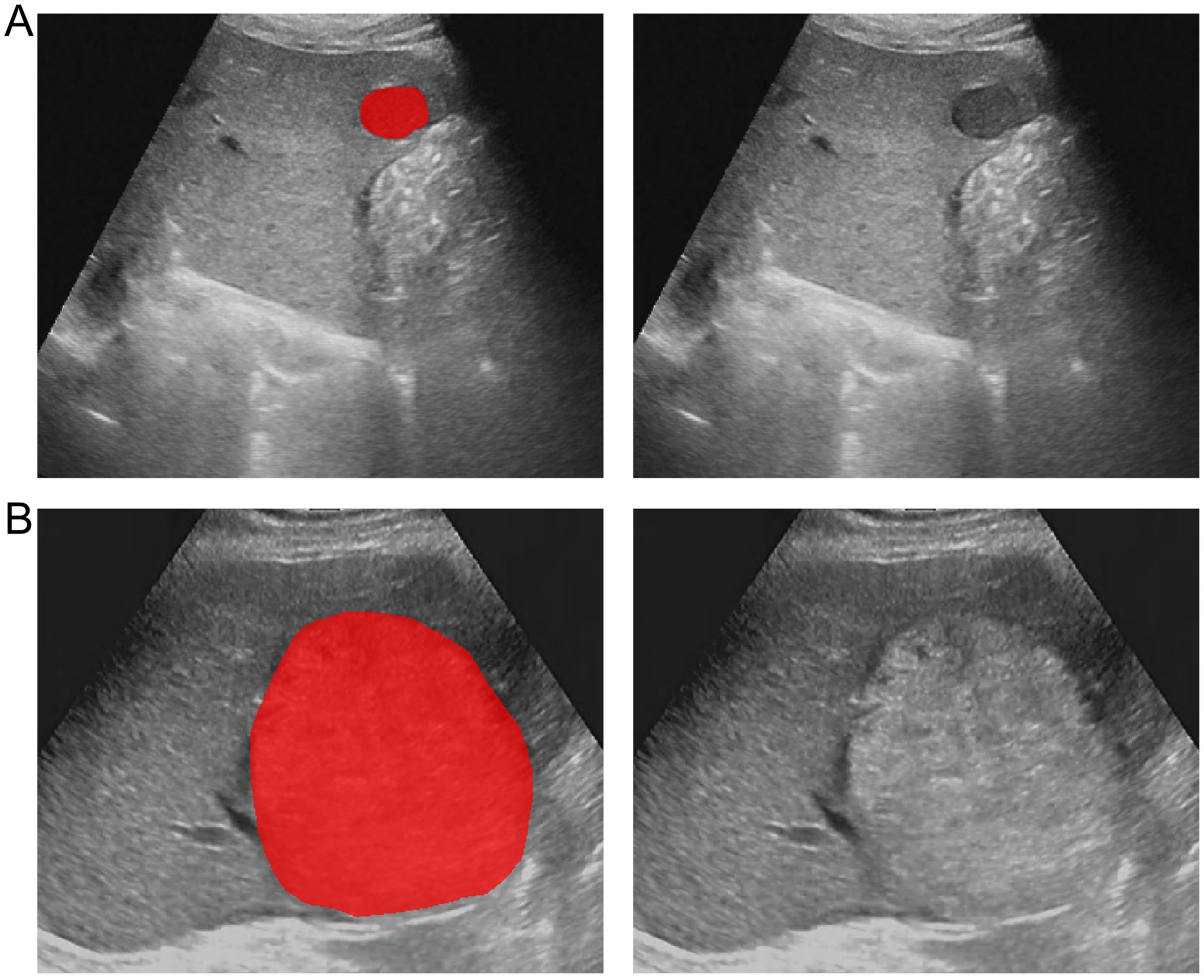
Example of delineating region of interest (ROI) on grayscale ultrasound images. (A) The lesion segmentation image and original image of a patient with wild-type *TP53*. (B) The lesion segmentation image and original image of a patient with mutant *TP53*.
